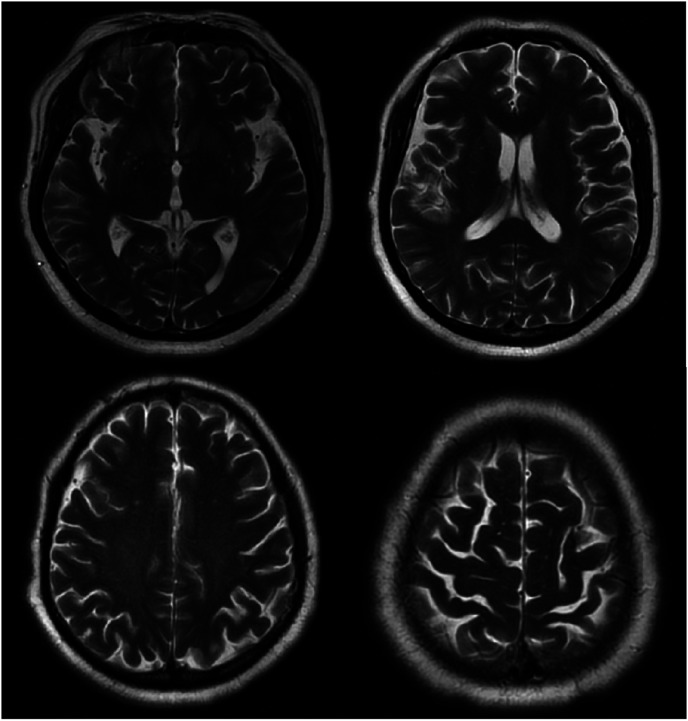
Magnetic resonance imaging of the brain, T2-weighted sequence, showing no significant abnormalities.
